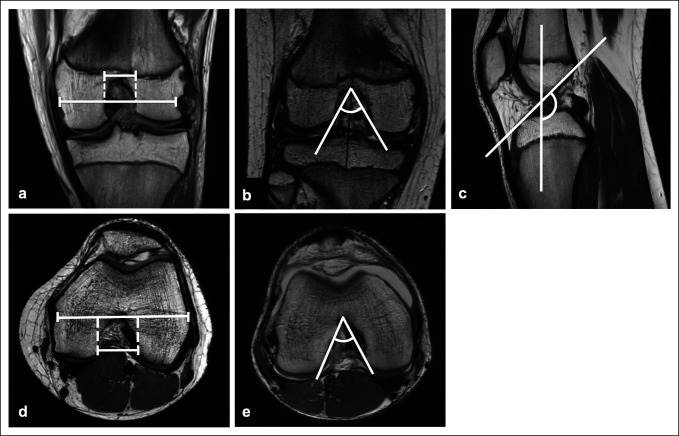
Radiographs showing that the notch width index is the ratio of the intercondylar notch width to the bicondylar width of the distal femur at the level of the popliteus groove in coronal (A) and axial (D) views. The notch angle is measured at the level of the popliteus groove, tracing the opening of the intercondylar notch in coronal (B) and axial (E) views. Roof inclination angle is the obtuse angle formed by the intersection of a line over the Blumensaat line and a line parallel to the long axis of the knee in the midsagittal view (C).
